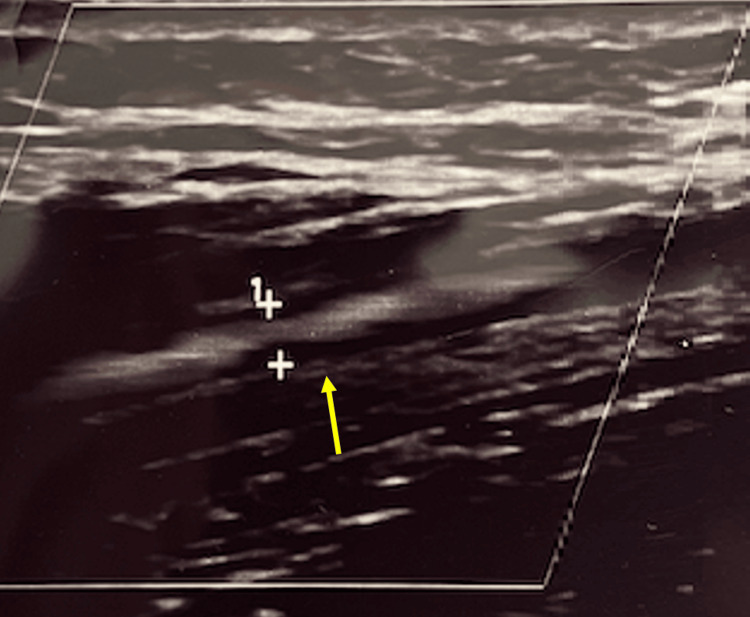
Post-LSB: the PTA measures .27 cm in diameter with a blood flow velocity of 57.4 cm/s (note Figure 1 and Figure 2 are not to scale and Figure 2 is with a Doppler) LSB: lumbar sympathetic block; PTA: posterior tibial artery
